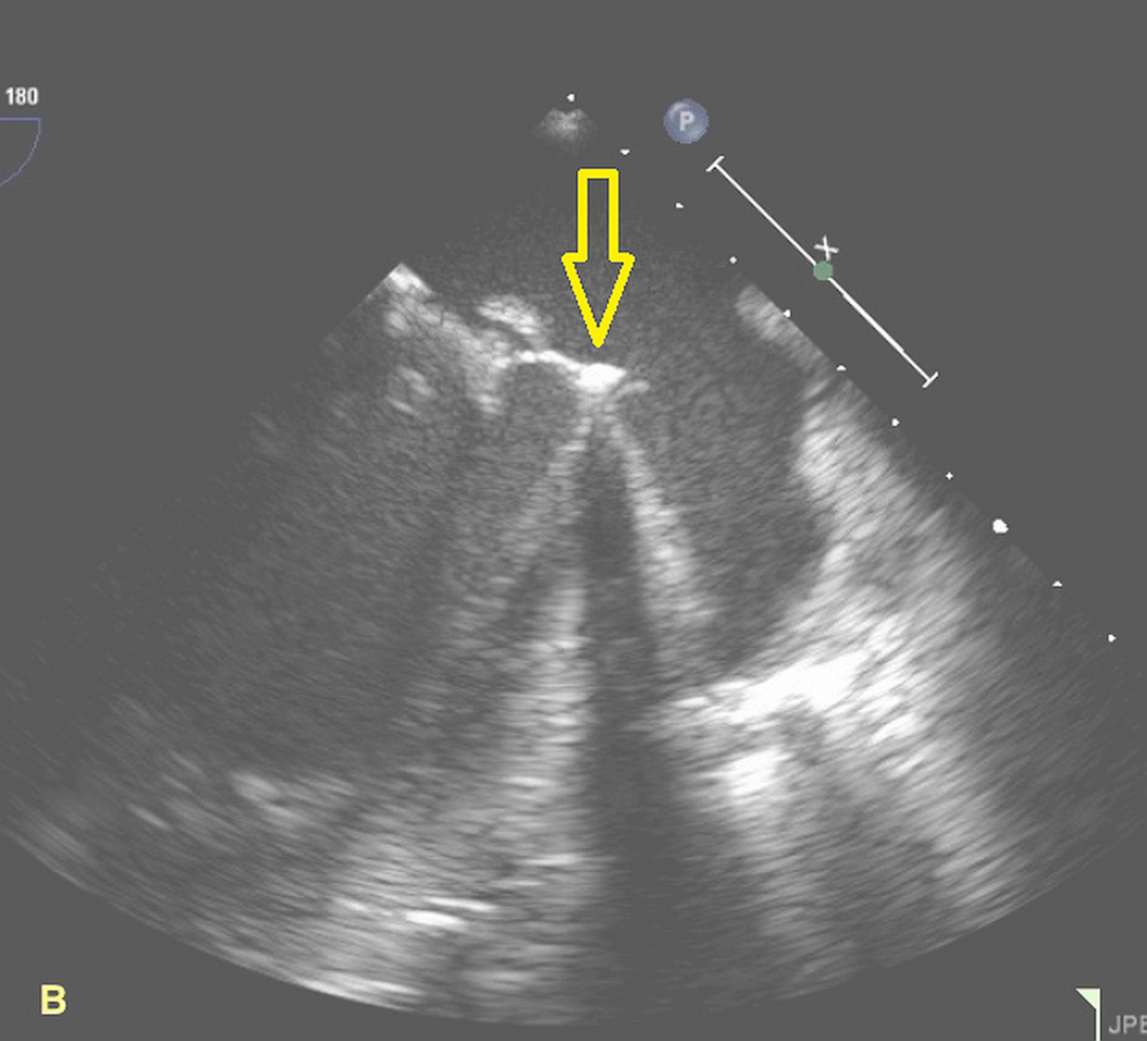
Two-dimensional echocardiography showing the prosthetic mitral valve along with its vegetations.
